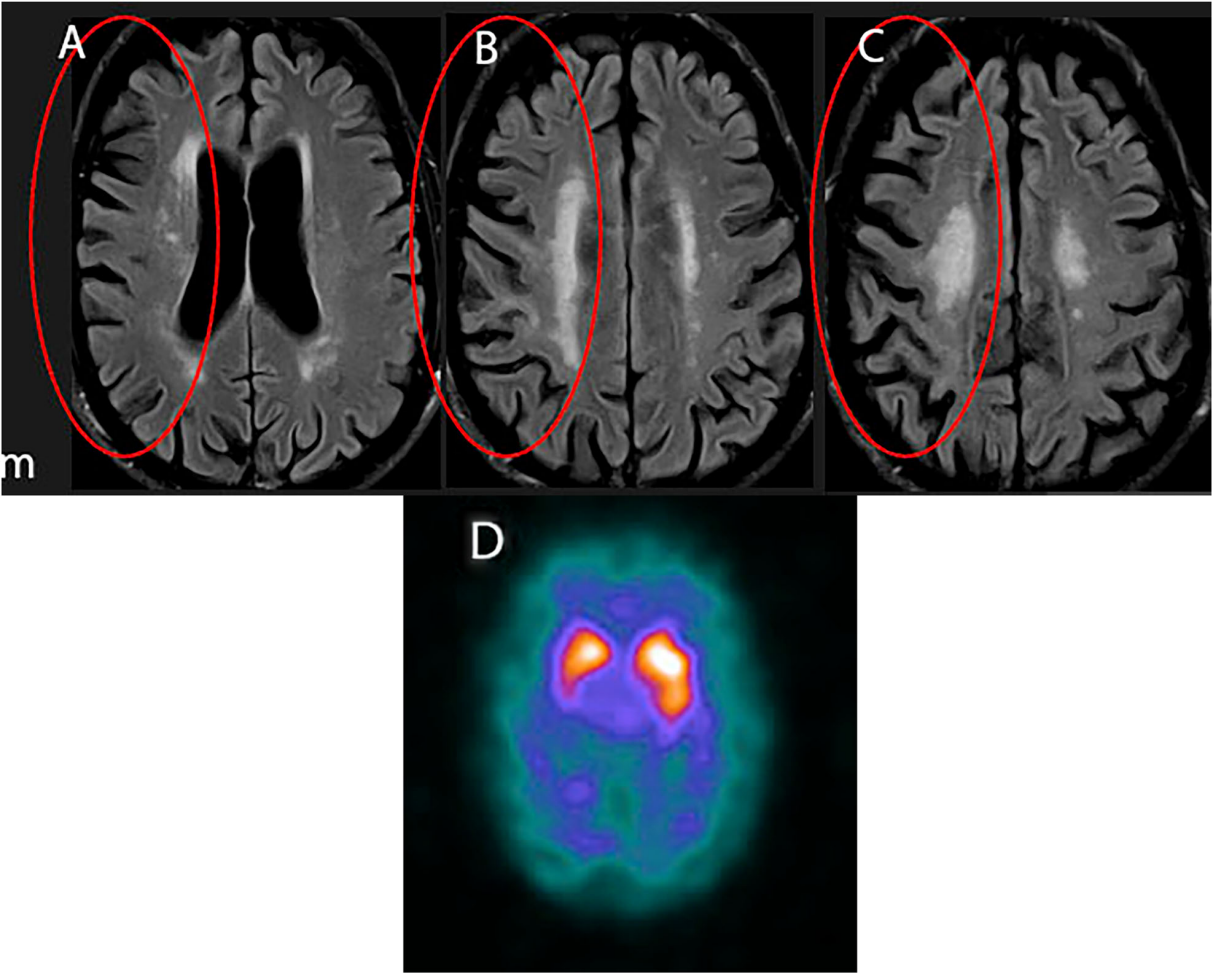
74-year-old male with tremor, rigidity, difficulty in using the left upper extremity, developed progressive nonfluent speech. MOCA reveals 18/30, unresponsive to levodopa. Executive function is preserved, and no personality changes are present. FLAIR axial images of the lateral ventricles (A), coronal radiata (B), and central semiovale (C) show profound asymmetric cortical volume loss over the right frontal and parietal cortex with asymmetric white matter T2 FLAIR hyperintensity, more in the right cerebral hemisphere. Dopaminergic receptor SPECT image (D) demonstrates asymmetric tracer reduction in the left striatum. The findings are consistent with corticobasal degeneration (CBD)
